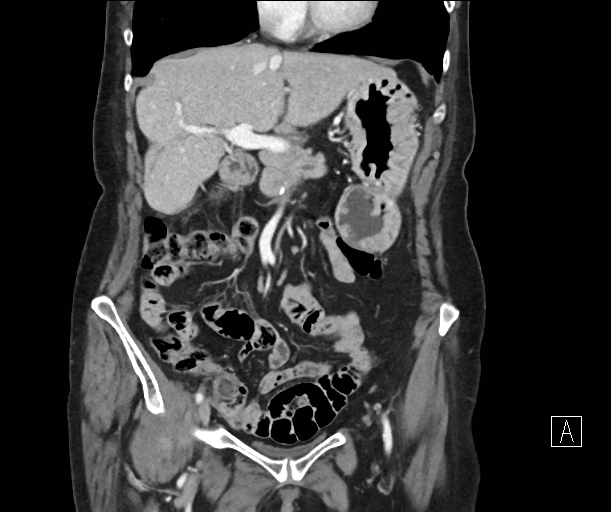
Coronal portal venous phase contrast-enhanced CT: complete resolution of the left upper quadrant collection.
